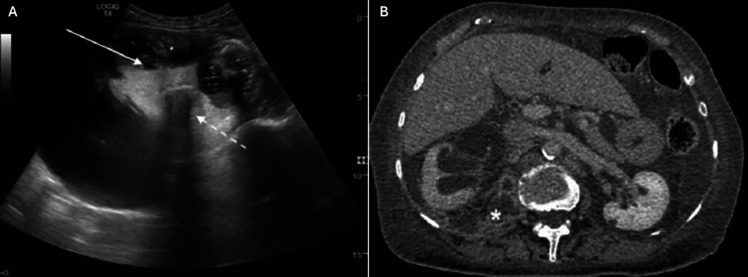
Radiological images of pyonephrosis. A US shows hydronephrosis and cortical thinning with high-grade dilation of the kidney collecting system filled with fluid and hyperechoic debris. B Axial CT imaging following intravenous contrast administration reveals hydronephrosis and thickening of the kidney pelvic wall. The perirenal space contains fluids, and an abscess is observed in the ileopsoas muscle. Adapted from reference [35], reproduced under Creative Commons License CC BY. Ultrasound, US; computed tomography, CT
